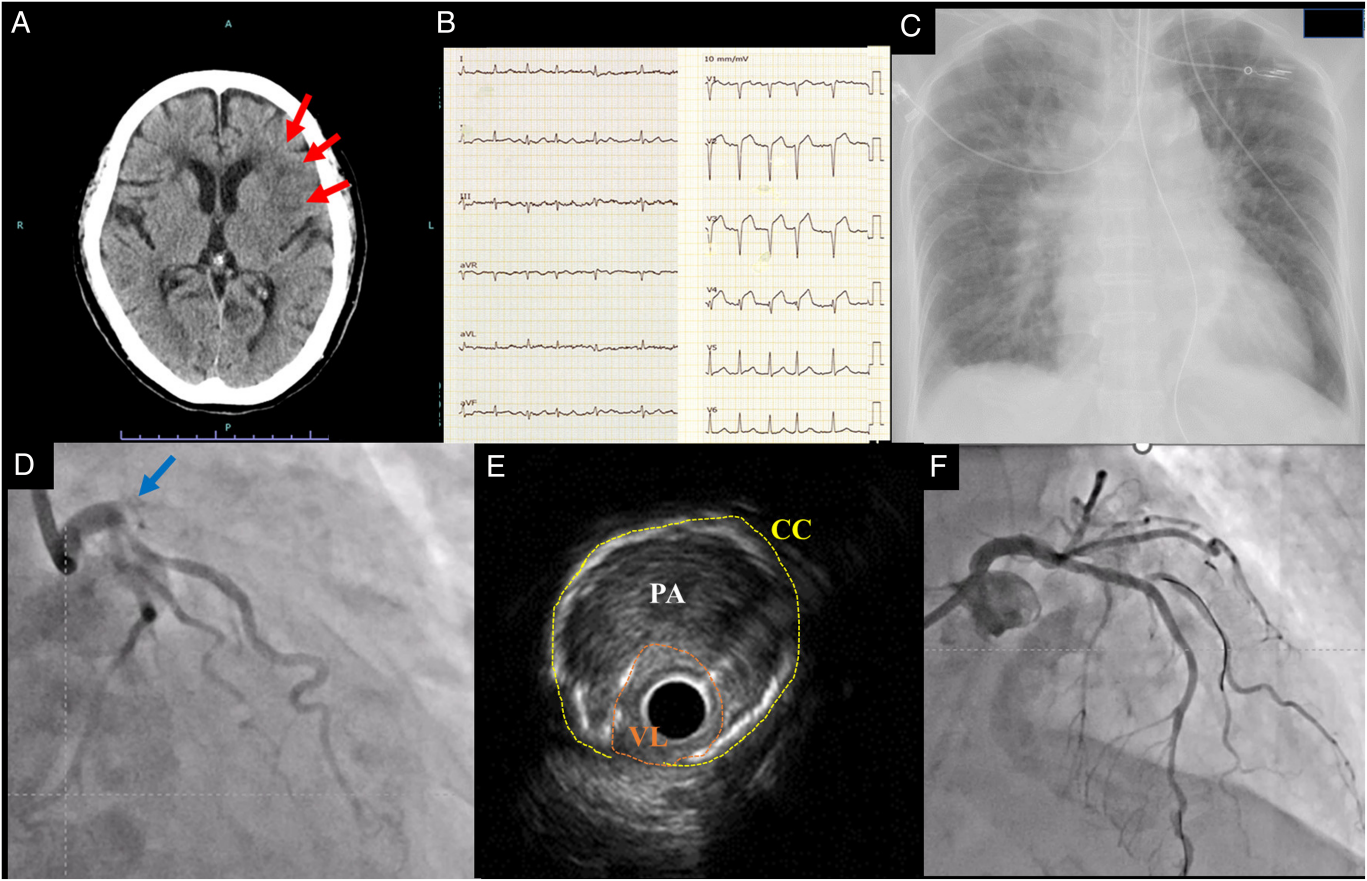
Representative case of cardio-cerebral infarction requiring coronary stenting for myocardial infarction. Head CT showed low density area in left middle cerebral artery area (red allows) at admission (A). Electro-cardiogram showed ST-segment elevation in anterior leads (B). Chest X ray showed pulmonary congestion (C). Coronary angiography showed total occlusion at proximal of left anterior descending artery (blue arrow) (D). Intravascular ultrasound showed that vessel lumen (VL) was narrowed (highlighted in orange) by plaque area (PA) and circumferential calcification (CC) (highlighted in yellow) due to atherosclerotic changes in the culprit lesion (E). TIMI 3 flow was obtained after stenting, and the stented lesion was indicated (F).
